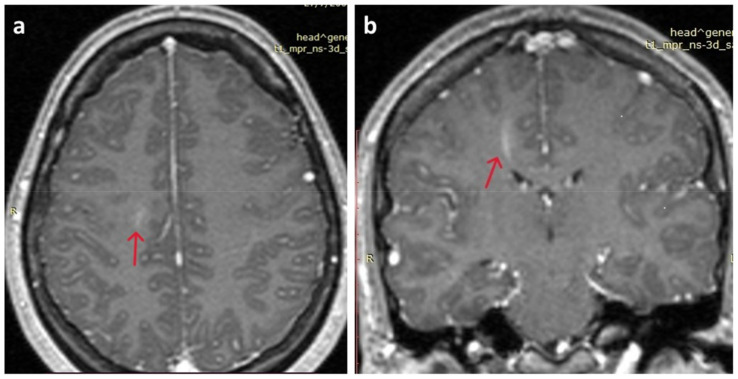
Brain MRI. (a) Mild leptomeningeal enhancement in both brain hemispheres (red arrow); (b) linear enhancing formation in the right centrum semiovale, characterized as a probable venous angioma S (red arrow).
